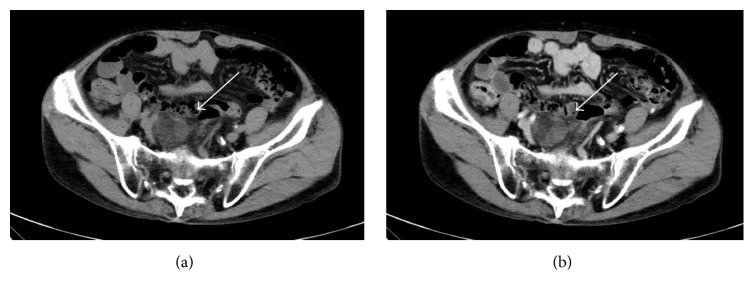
Enhanced CT scan showed a heterogenous round mass in the presacral space (diameter: 43 mm) that contained low-density areas ((a) plane, (b) arterial phase).
